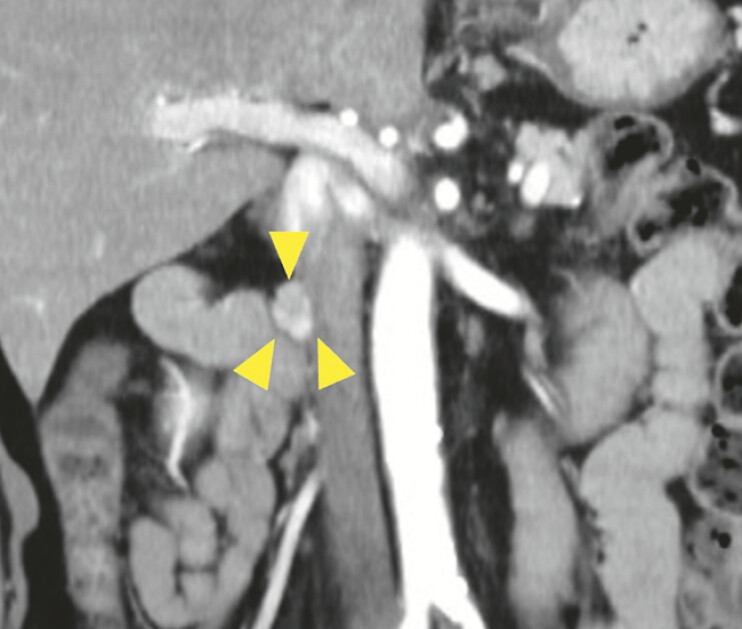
Computed tomography image of a recurrent lymph node lesion (arrowheads) of the retroperitoneal paraganglioma.
